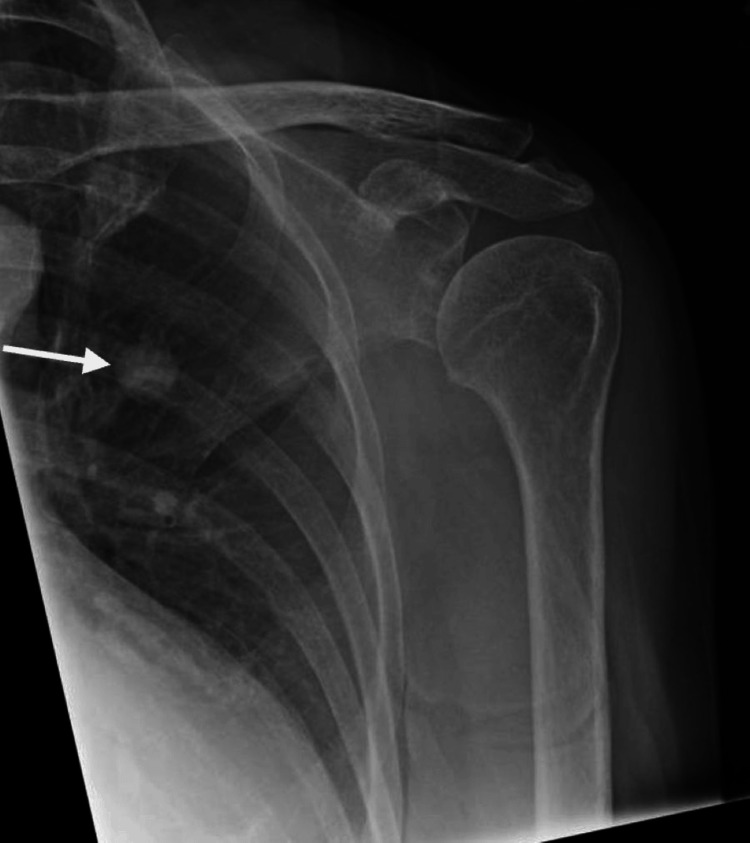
Left shoulder X-ray showing a 16 mm pulmonary nodule (arrow)
